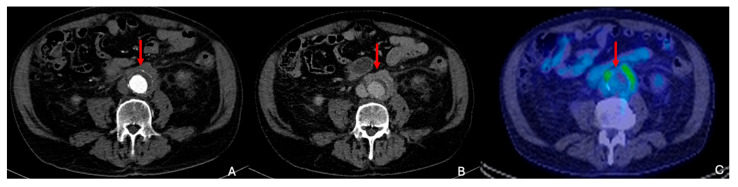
Inflammatory AAAs. (A) Arterial phase CTA imaging demonstrates an infrarenal AAA measuring 3.5 cm, accompanied by visible periaortic tissue (red arrow). (B) The periaortic tissue (red arrow) exhibits enhancement in the delayed phase of CTA, commonly referred to as the “mantle sign”. (C) Fused PET-CT images reveal significant ^18^F-FDG uptake within the periaortic tissue (red arrow). Subsequent evaluation identified the inflammatory aneurysm as being associated with IgG4-related disease.
